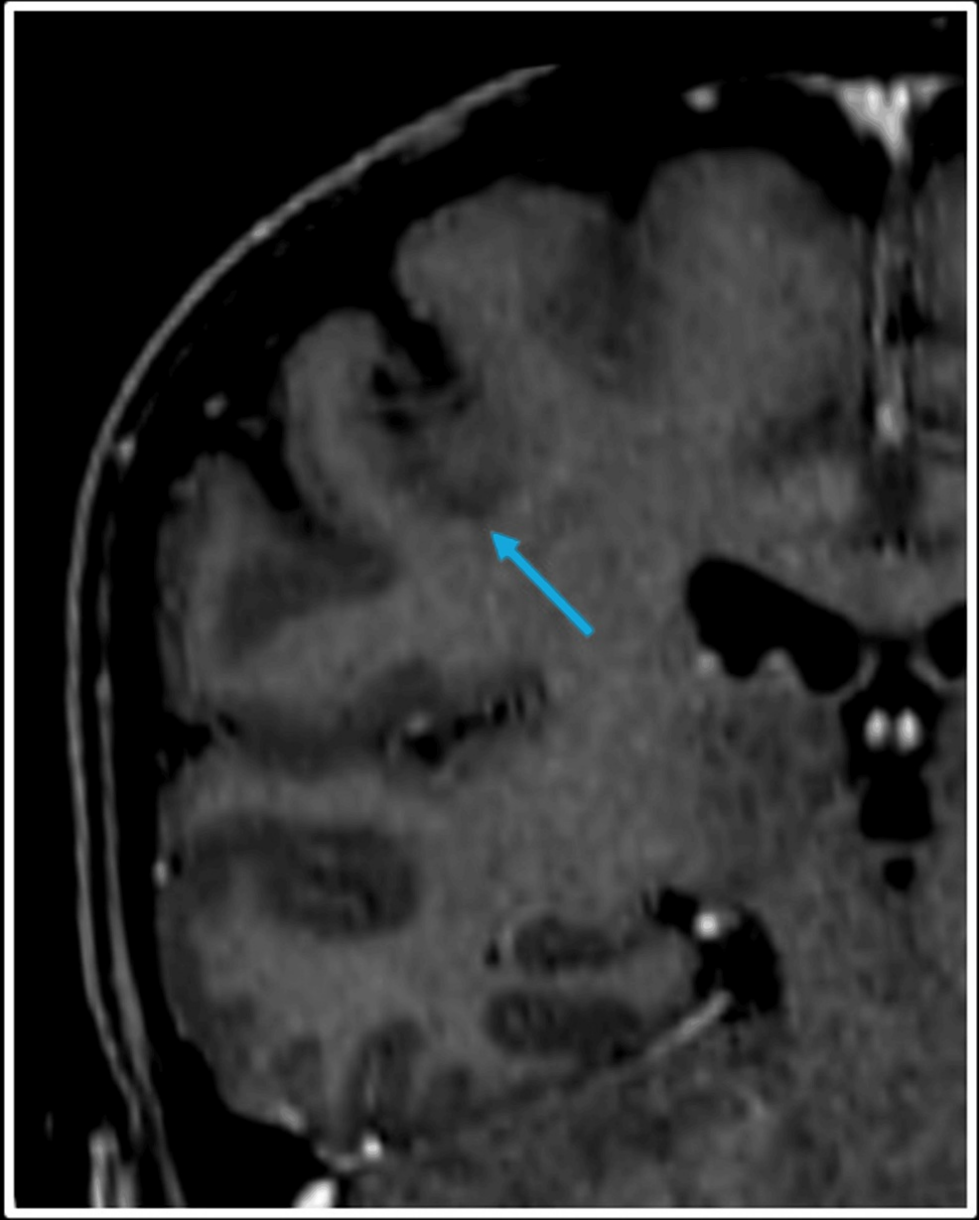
Coronal post-Gadolinium T1-weighted MRI The image shows the resolution of the previously seen non-irradiated lesion at the grey-white matter junction inferior to the right central sulcus (blue arrow).
